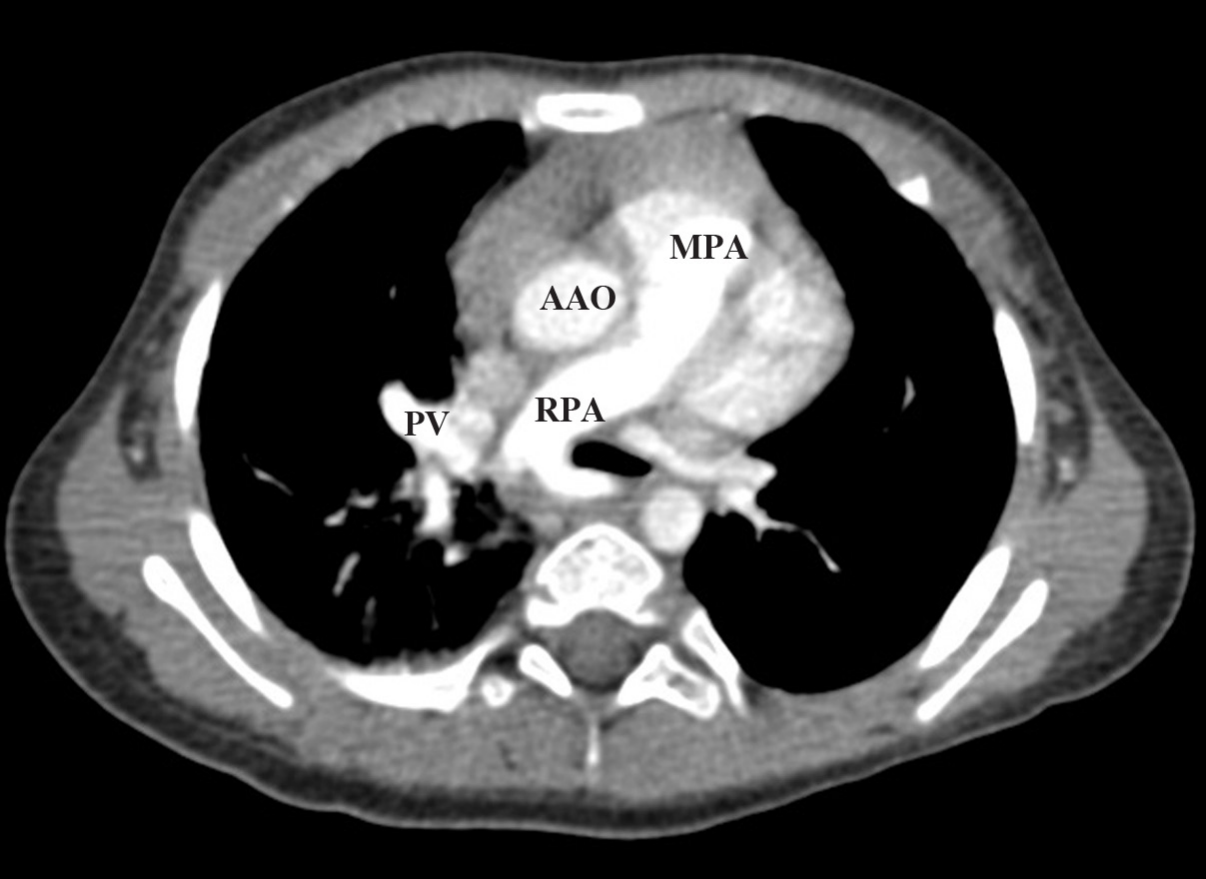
Computed tomography angiography scan of the thorax, showing pulmonary artery sling.
